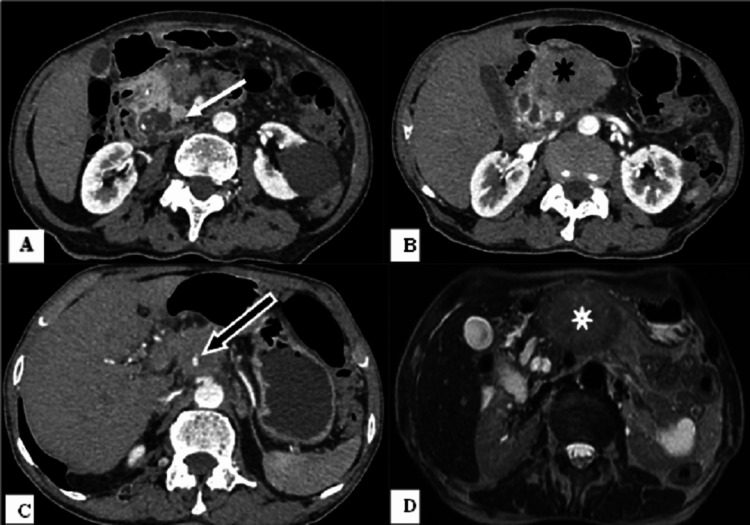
Chronic pancreatitis and carcinoma pancreas in a case of partial agenesis of the dorsal pancreas. Contrast-enhanced computed tomography of the abdomen (A) showing calcific foci with dilated duct (white arrow) in the head of the pancreas. An ill-defined heterogeneously hypoenhancing mass (B, black star) is seen in the visualized region of the body of the pancreas encasing the celiac trunk (C, black arrow). Axial T2-weighted imaging (D, white star) showing the mass in the body of the pancreas with non-visualization of the body and tail of the pancreas.
